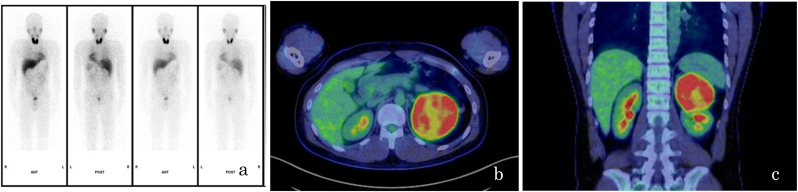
Imaging findings. (a) ^123^I-meta-iodobenzylguanidine scintigraphy showing no signs of a pheochromocytoma. (b, c) F-18 fluorodeoxyglucose positron emission tomography/computed tomography revealing strong uptake in the left adrenal region with a maximum standardized uptake value of 21.39.
